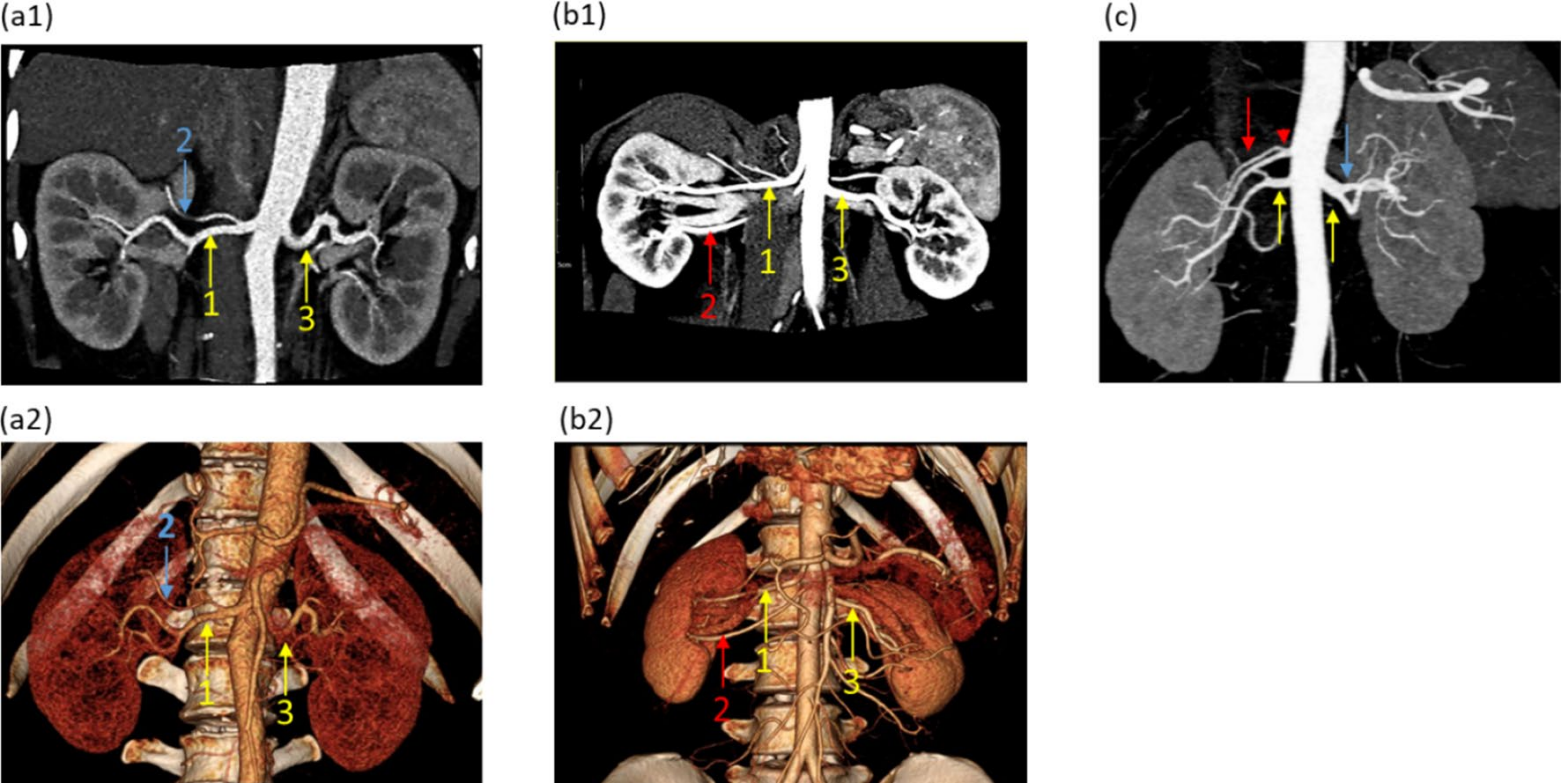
Multi-detector computed tomography angiography of the renal artery. (a) Main renal arteries and an early branching renal artery. (b) Main renal arteries and an accessory renal artery. The same numbers in (a1, b1) indicate the same arteries in (a2, b2). (c) A branching accessory renal artery (red arrowhead), main arteries, an early branching artery. The main, accessory, and early branching arteries are yellow, red, and blue arrows, respectively.
